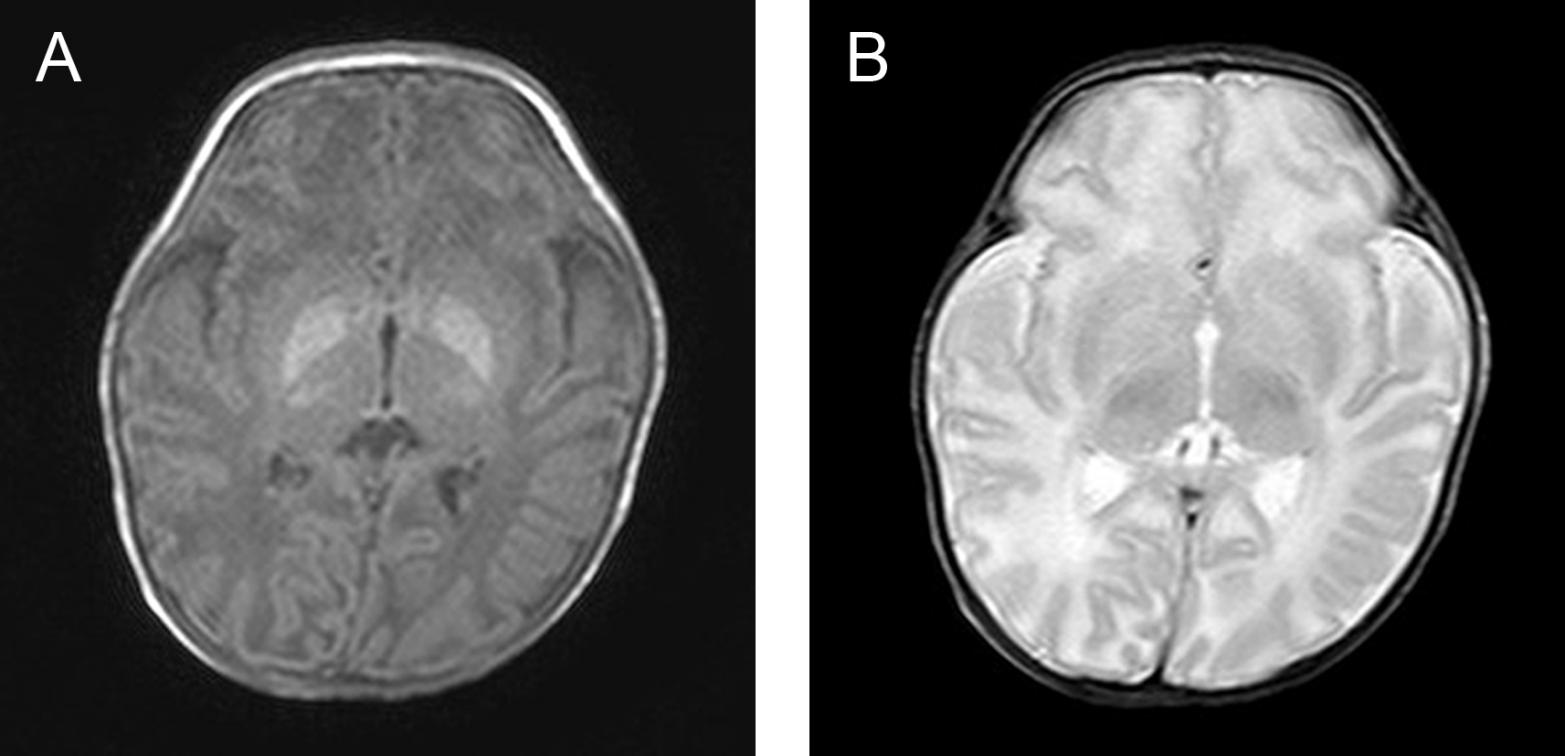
T1 and T2 weighted MR imaging at day 7 (patient number 21). A: There was bilateral, symmetric, abnormal increased signal intensity in the GP on T1-weighted imaging. B: The above-mentioned lesions were not apparent on T2-weighted images.
